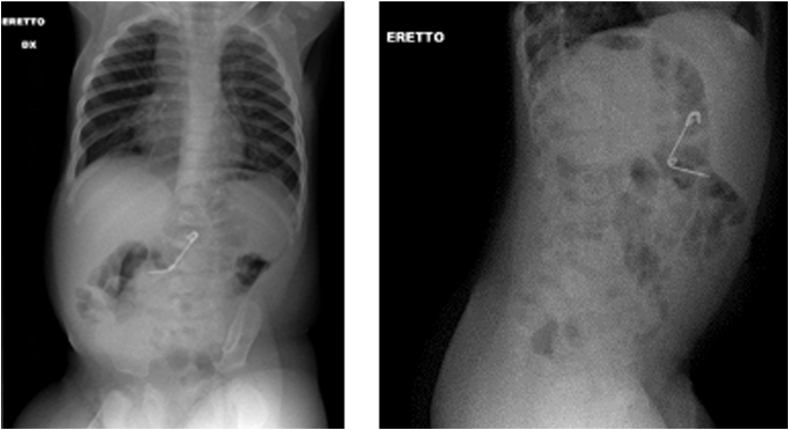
Anteroposterior (on the right) and lateral (on the left) X-ray of the thorax and abdomen.
